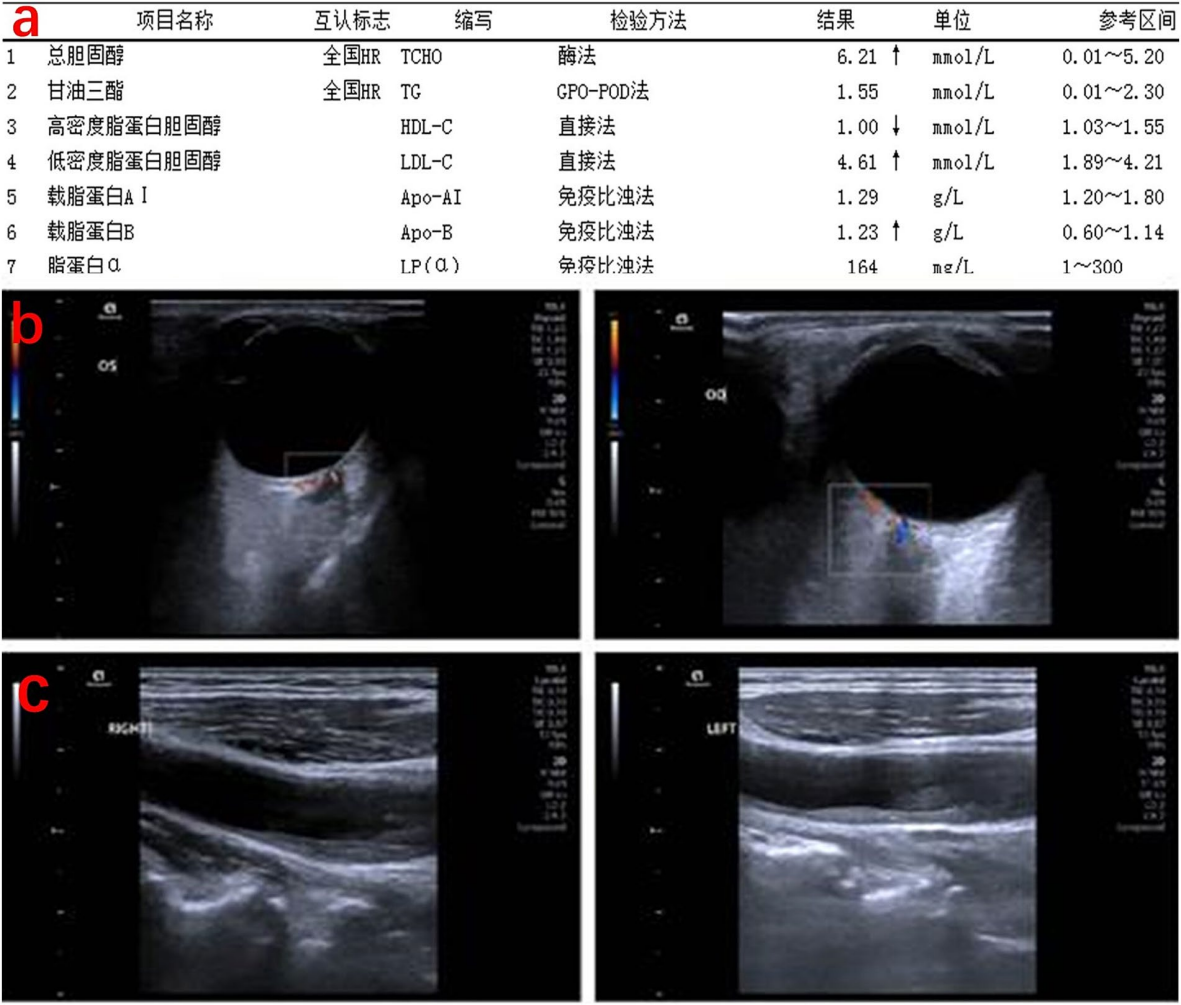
Lipid profile and vascular status of the patient. (a) Lipid profile: total cholesterol significantly elevated. (b) Posterior ocular vessel ultrasound: the right retinal central artery shows significantly reduced flow and velocity, with occlusion not ruled out. Reduced flow velocity in the right nasal ciliary posterior short artery. (c) Carotid ultrasound: bilateral carotid artery walls are irregular, with hypoechoic, hyperechoic, and mixed echogenic plaques visible in the common carotid artery, bifurcation, and the origin of the internal carotid artery. Blood flow filling defects are observed at the plaque sites.
